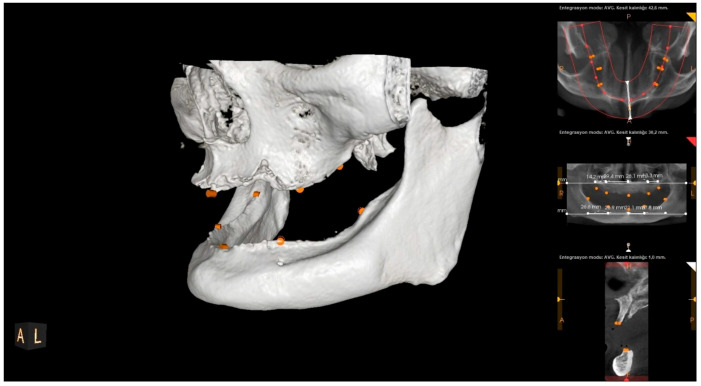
Landmarks on a CBCT image (left side view).
